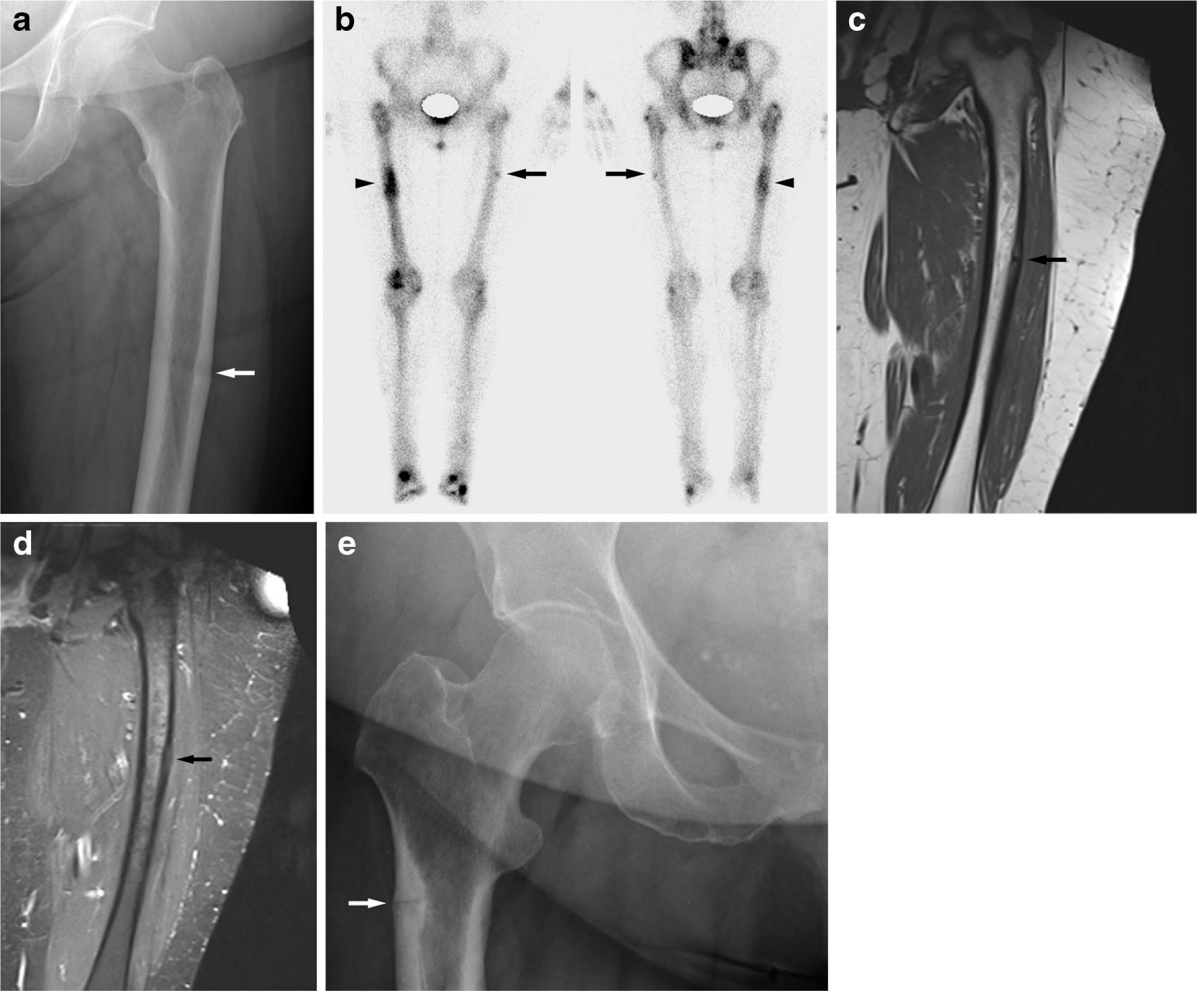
An elderly female on long-term bisphosphonates for osteoporosis had suffered a sudden fracture of the right femoral shaft after a period of niggling right thigh pain. This had been treated with an intramedullary nail. Then similar pain developed on the left side. A radiograph (a) shows subtle cortical thickening of the lateral aspect of the femoral shaft (see arrow). Bone scintigraphy (b) and MRI performed 1 month later (coronal T1w c, coronal STIR d) show mild uptake of the left femoral shaft and a minor bone reaction of the left lateral femoral shaft (see arrows). Marked uptake of the right femoral shaft on the bone scan is due to previous fracture; an intramedullary nail is in situ resulting in increased uptake in the proximal femur (see arrowhead). This is a so-called atypical femoral fracture. An AP radiograph of the hips performed for right hip/groin pain in another elderly female patient shows a more advanced case with a fracture through the lateral subtrochanteric femoral shaft cortex with a minor bone reaction; only a small bony spike is seen (e, arrow). Atypical femoral shaft fractures often affect elderly patients on long-term bisphosphonates. The lateral femoral cortex is affected first, bone reaction is minor, and the fracture lines are fairly horizontal
